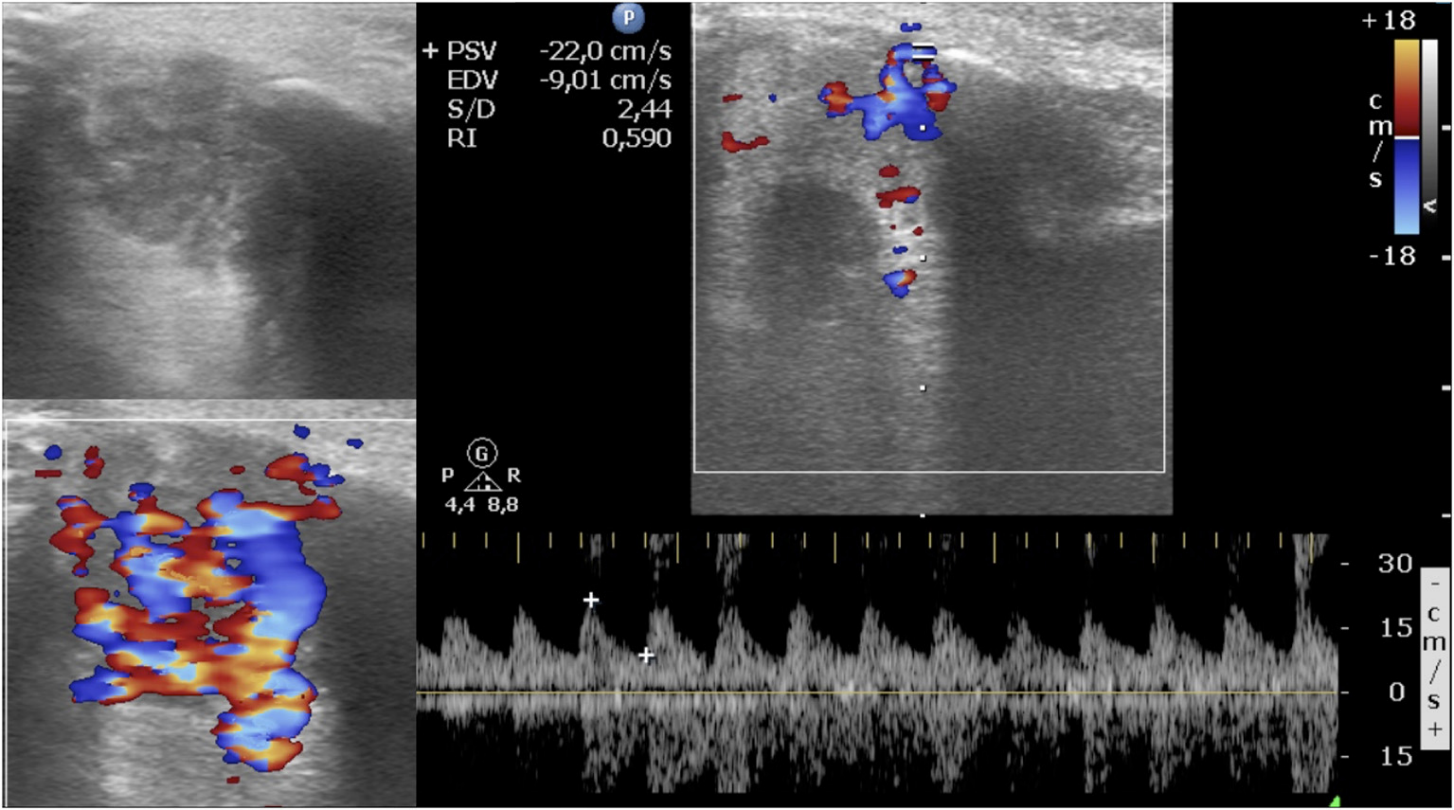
Periorbital infantile hemangioma. Baseline ultrasound images show a circumscribed mostly hypoechoic mass; at color Doppler, the lesion is highly vascularized, and the resistive index is 0.59.
